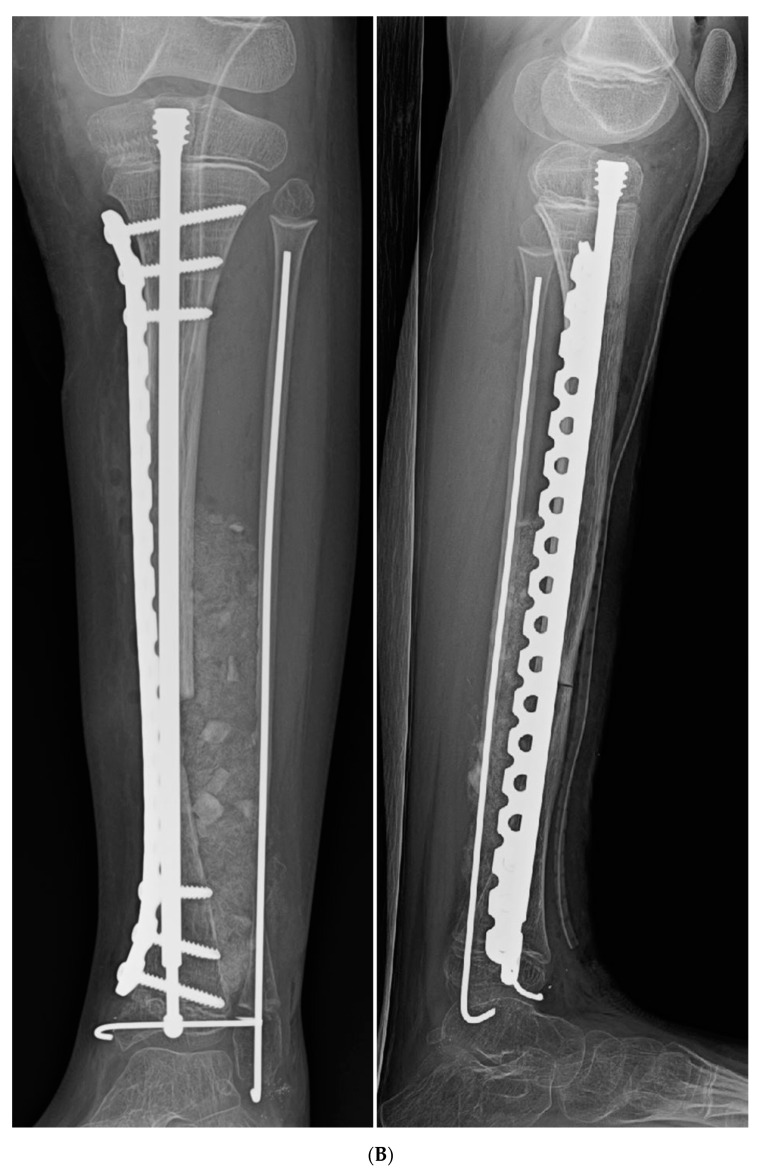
(A) AP and lateral radiographs that were taken postoperatively of a patient who underwent a Paley cross-union procedure performed with a circular external fixator. (B) AP and lateral radiographs that were taken postoperatively of a patient who underwent a Paley cross-union procedure performed with all internal fixation.
